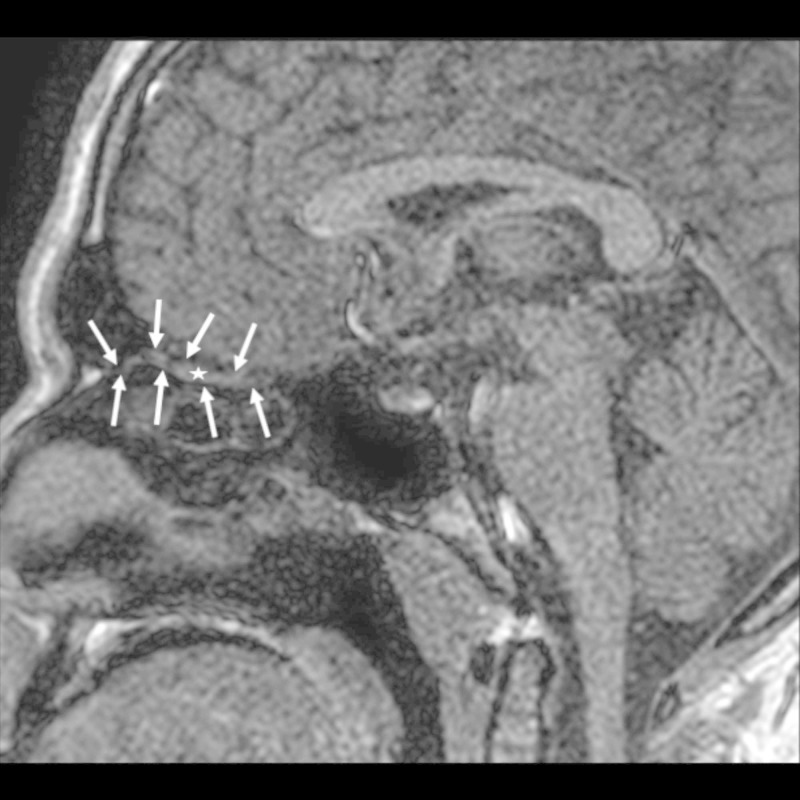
Sagittal T1-weighted image Sagittal T1-weighted image showing the boundaries of the left olfactory tract (arrows). The midsection of the olfactory tract is indicated by an asterisk.
